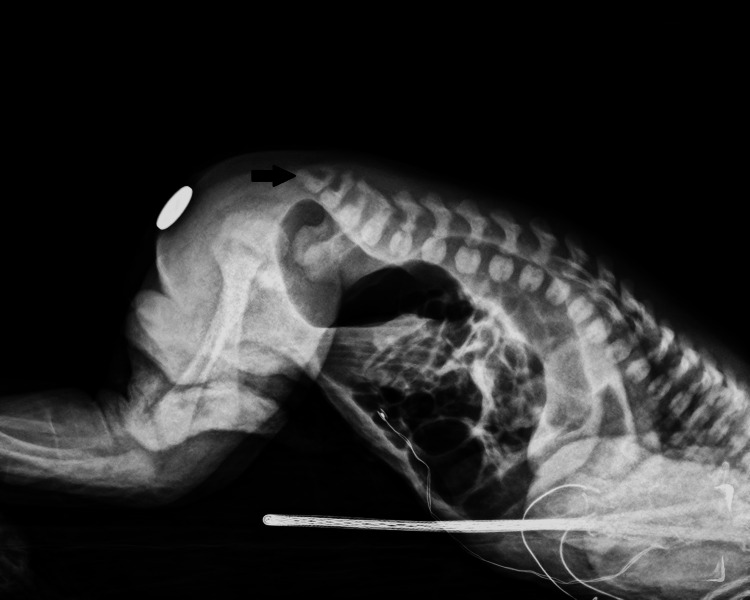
Spine radiograph Spine X-ray showing an apparently well-formed sacrum (black arrow), without pre-sacred masses visualized.
